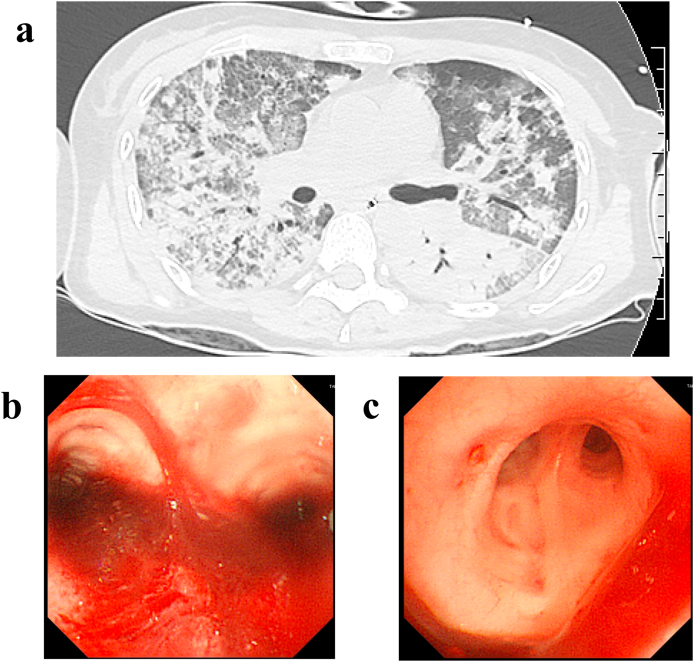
Imaging findings upon admission to our intensive care unit. (a) Computed tomography revealed diffuse consolidation and atelectasis due to diffuse alveolar hemorrhage affecting both lungs. (b) Bronchoscopy of the carina showing diffuse hemoptysis. (c) Bronchoscopy of the right upper bronchus showing an ulcer and hemoptysis.
